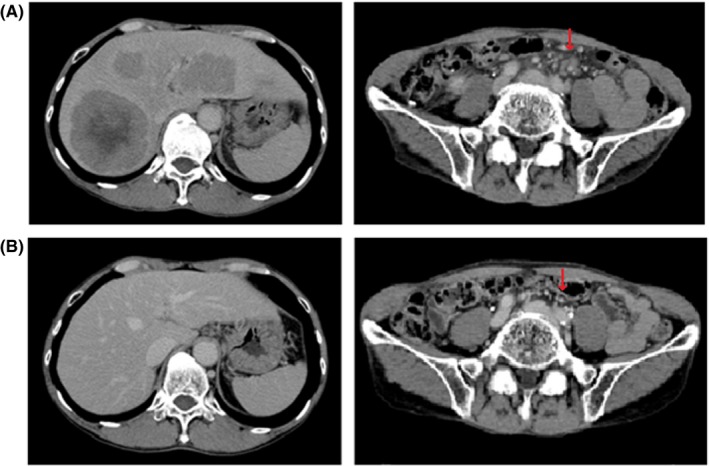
Enhanced computed tomography scan findings in the case 1. A, Multiple liver metastases in the both lobes and enlarged lymph nodes along the branch of superior mesenteric artery region (arrow) were identified before chemotherapy. B, Remarkable response of liver metastases and lymph node metastases were maintained using trastuzumab plus capecitabine therapy
